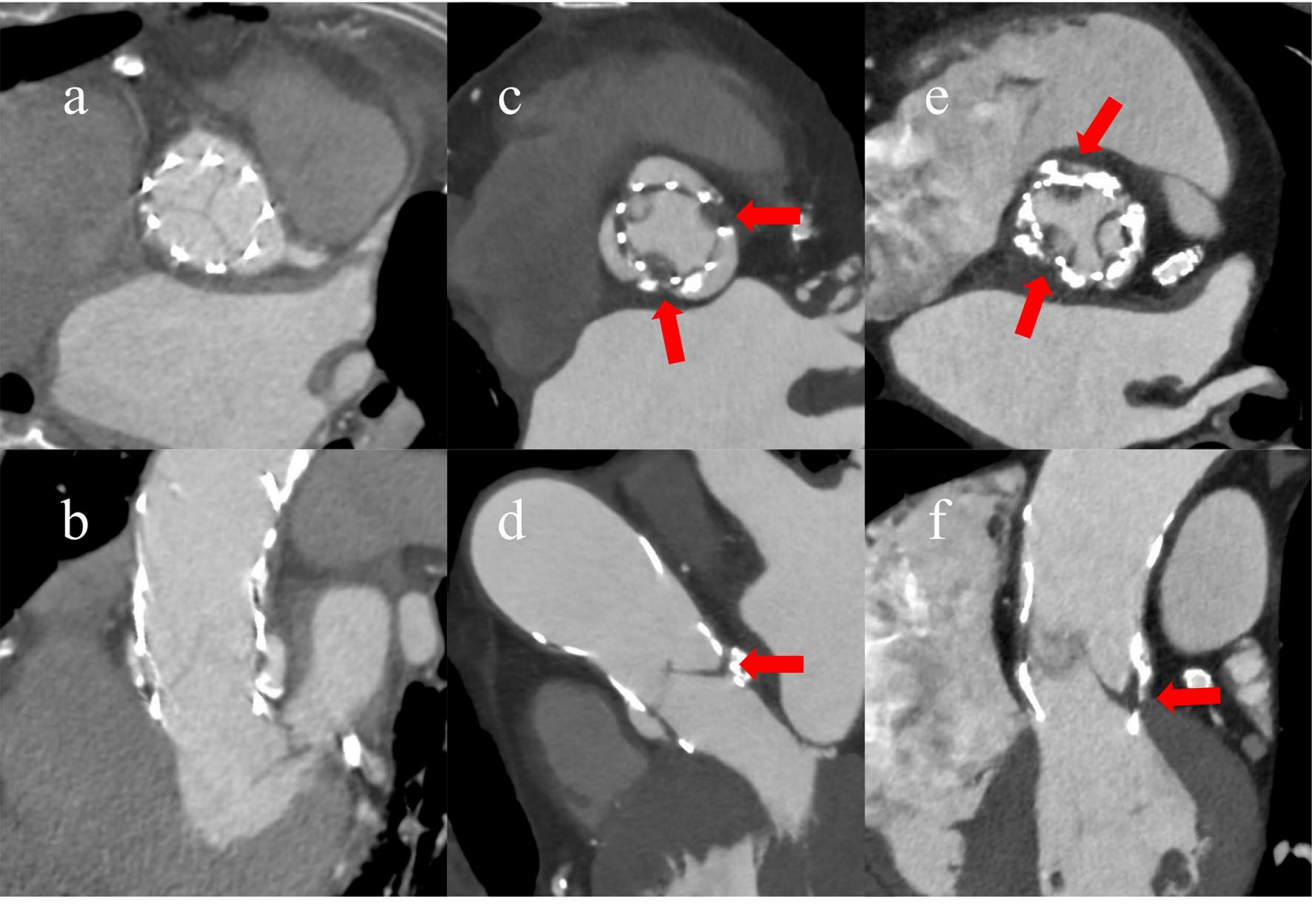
Representative cases of HALT. Contrast-enhanced CT images after transcatheter aortic valve implantation showing axial reconstructions in end-systole or mid-diastole and corresponding coronal or sagittal oblique views. a, b Views without HALT. c, d Views with grade 2 HALT. e, f Views with grade 3 HALT. Red arrows indicate the presence of HALT. *HALT* hypo-attenuated leaflet thickening, *CT* computed tomography
